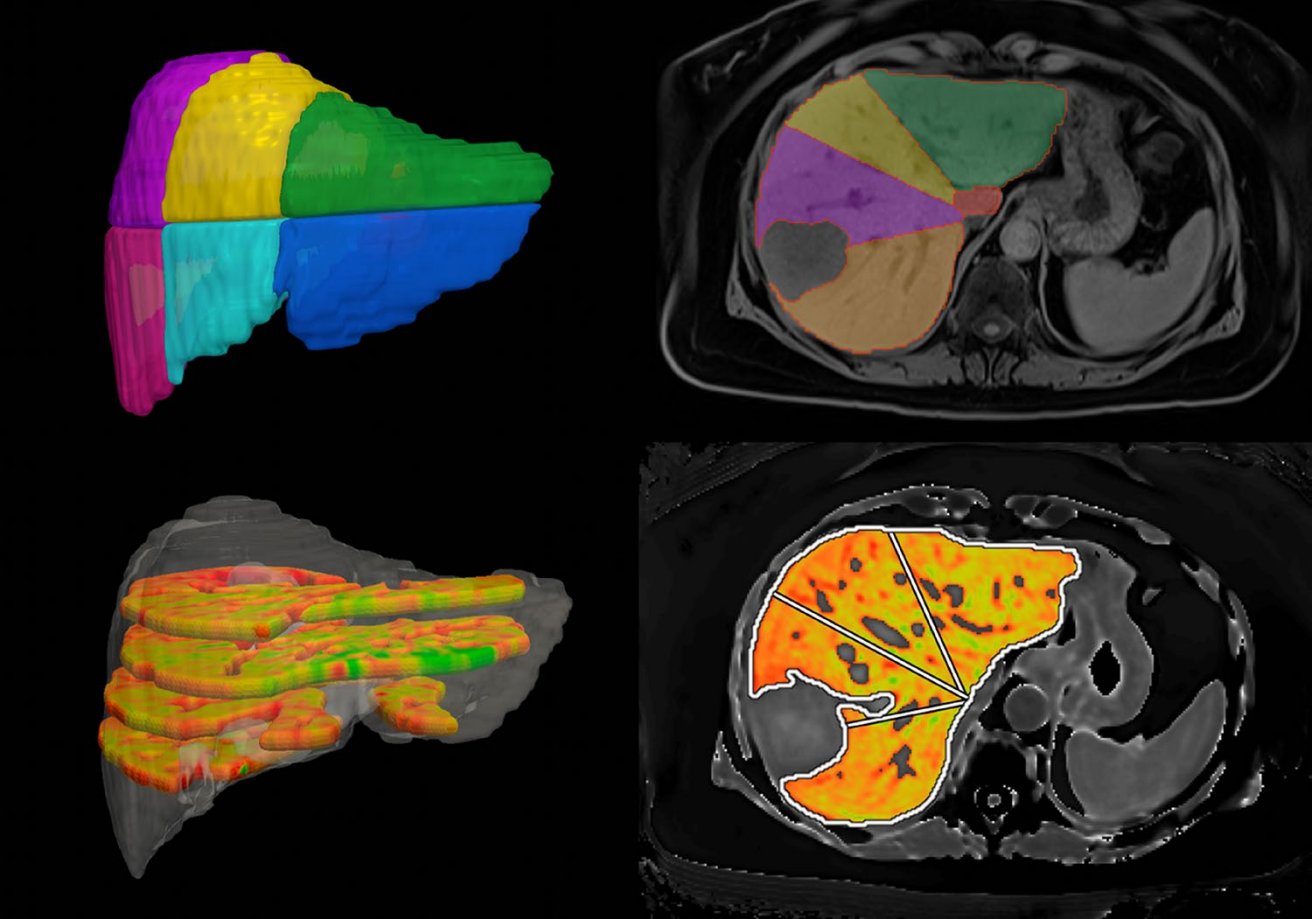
Example of Couinaud segment delineations from Hepatica™ on non-contrast-enhanced T1-weighted MR and cT1 images
